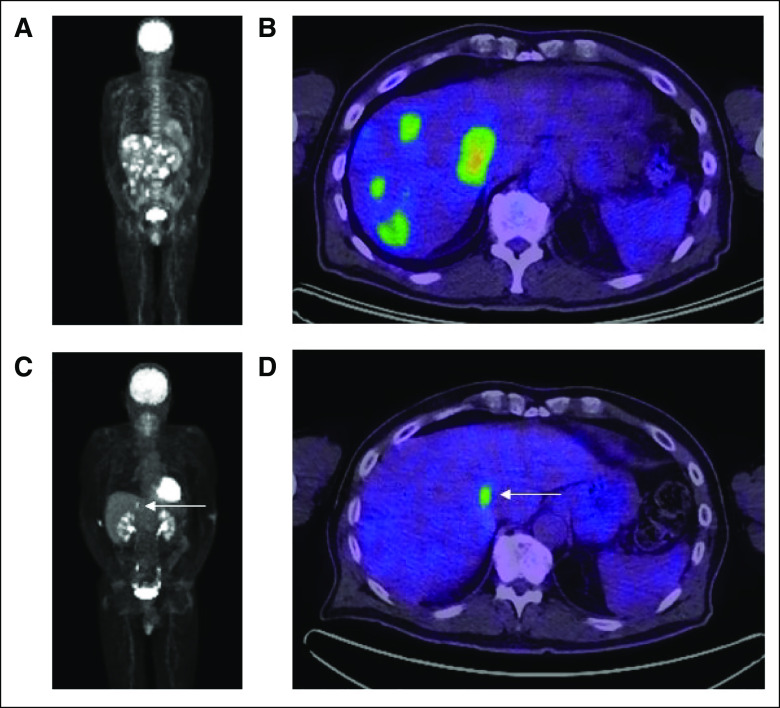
PET scan at baseline and after six cycles of carboplatin and nab-paclitaxel chemotherapy reveals excellent partial response to initial systemic therapy. Baseline PET scan including (A) MIP and (B) computed tomography fused axial views demonstrates pancreatic primary and extensive liver metastases. Follow-up (C) MIP and (D) fused axial views after six cycles of platinum-based chemotherapy with the addition of pembrolizumab from C4 demonstrate near-complete resolution of disease, with only a solitary remaining fluorodeoxyglucose-avid liver metastasis (arrow). MIP, maximum intensity projection; PET, positron emission tomography.
